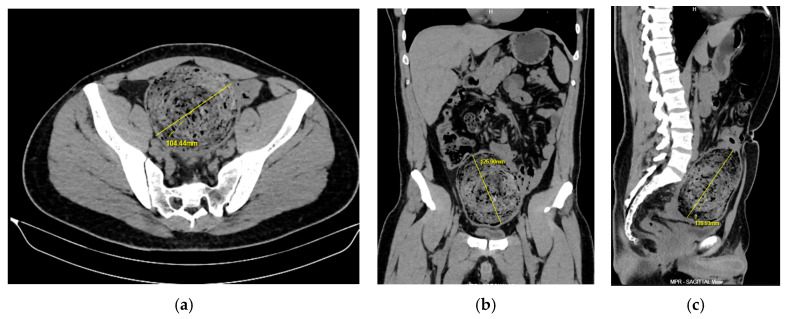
Computed tomography abdomen/pelvis in (a) axial plane, (b) coronal plane, (c) sagittal plane. Sigmoid colonic dilation with 11 cm large fecal mass.
